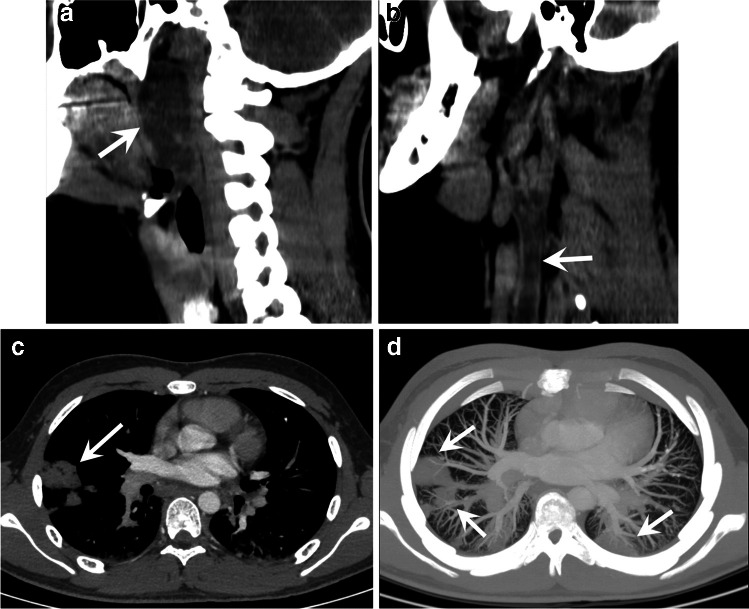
A 16-year-old boy with a peritonsillar abscess, trismus, and hypoxemia. A cervicothoracic contrast-enhanced computed tomography (CT) scan was performed. Sagittal multiplanar reconstruction reformatted images show peritonsillar abscess (*arrow in*
